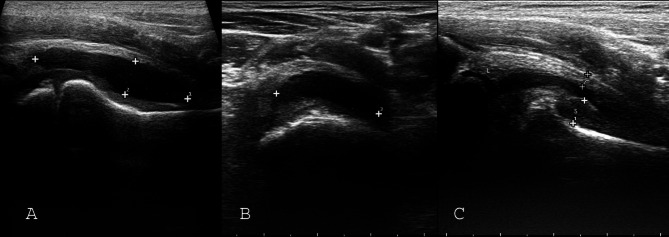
The sonographic measurements of hip effusion in anterior recess: (A) Longitudinal diameter and thickness of hip effusion. (B) Transverse diameter of anterior recess. The anterior recess volume was measured with these three dimensions. (C) Capsule thickness at the midpoint of the anterior recess and synovial thickness at the maximal point. Synovial thickening in reactive arthritis is obvious. L: Labrum, S: Synovium
